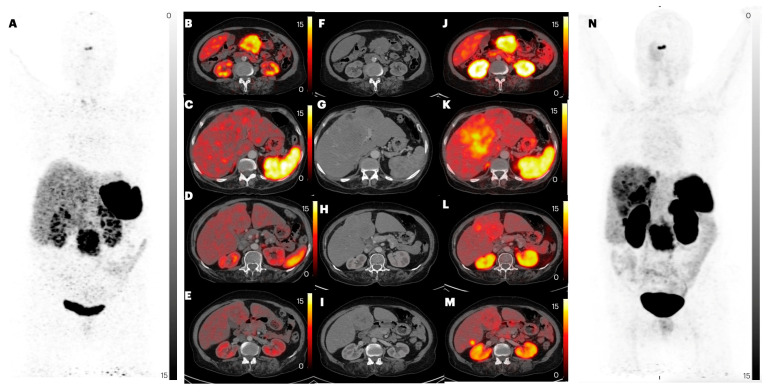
PET/CT images of patient number 16, a 64-year-old female with a known case of gastric NET with liver metastases with no treatment started and for whom IV contrast was given. Images (A,N) are MIP images of [^68^Ga]Ga-DOTANOC and [^68^Ga]Ga-DATA^5m^-LM4 PET/CT, respectively. Images (B,C,D,E) are [^68^Ga]Ga-DOTANOC PET/CT axial images. Images (F,G,H,I) are CT images. Images (J,K,L,M) are [^68^Ga]Ga-DATA^5m^-LM4 PET/CT images. Liver metastases with tracer uptake are noted in [^68^Ga]Ga-DATA^5m^-LM4 PET/CT images.
